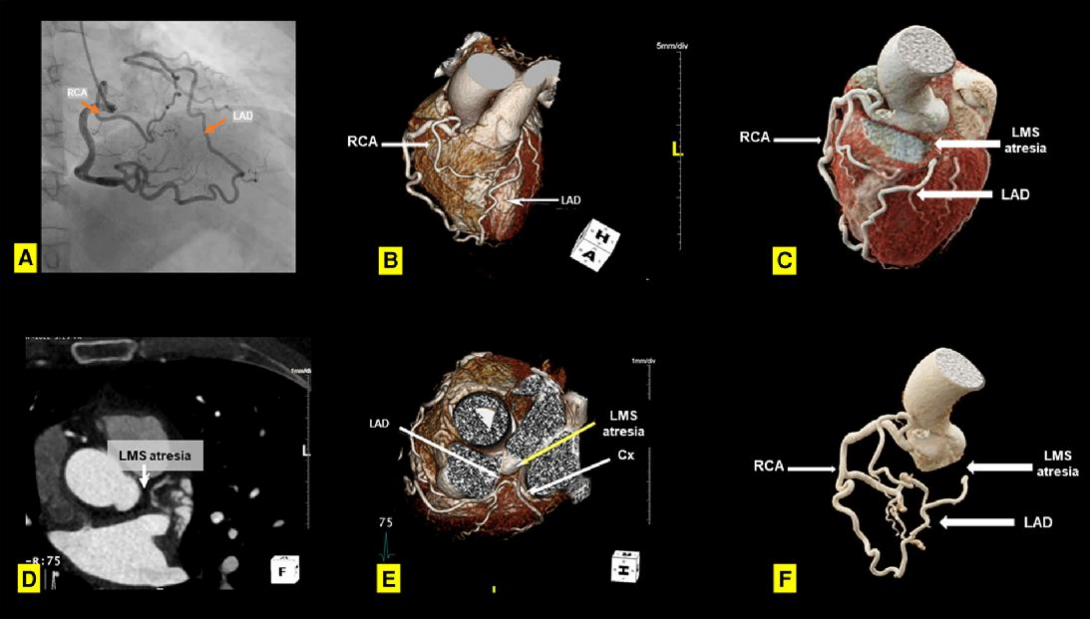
(*A*) Invasive coronary angiography. Right coronary catheterization showing left main stem atresia with collaterals from the right coronary artery supplying retrogradely the left coronary circulation. (*B–F*) Computed tomography coronary angiography. (*B* and *C*) 3D reconstruction showing collaterals between left anterior descending and right coronary artery and left main stem atresia. (*D*) 2D axial plane showing left main stem atresia. (*E*) 3D reconstruction showing left main stem atresia. (*F*) 3D reconstruction of the coronary tree showing collaterals between left anterior descending artery and right coronary artery and left main stem atresia. Cx, circumflex artery; LAD, left anterior descending artery; LMS, left main stem; RCA, right coronary artery.
